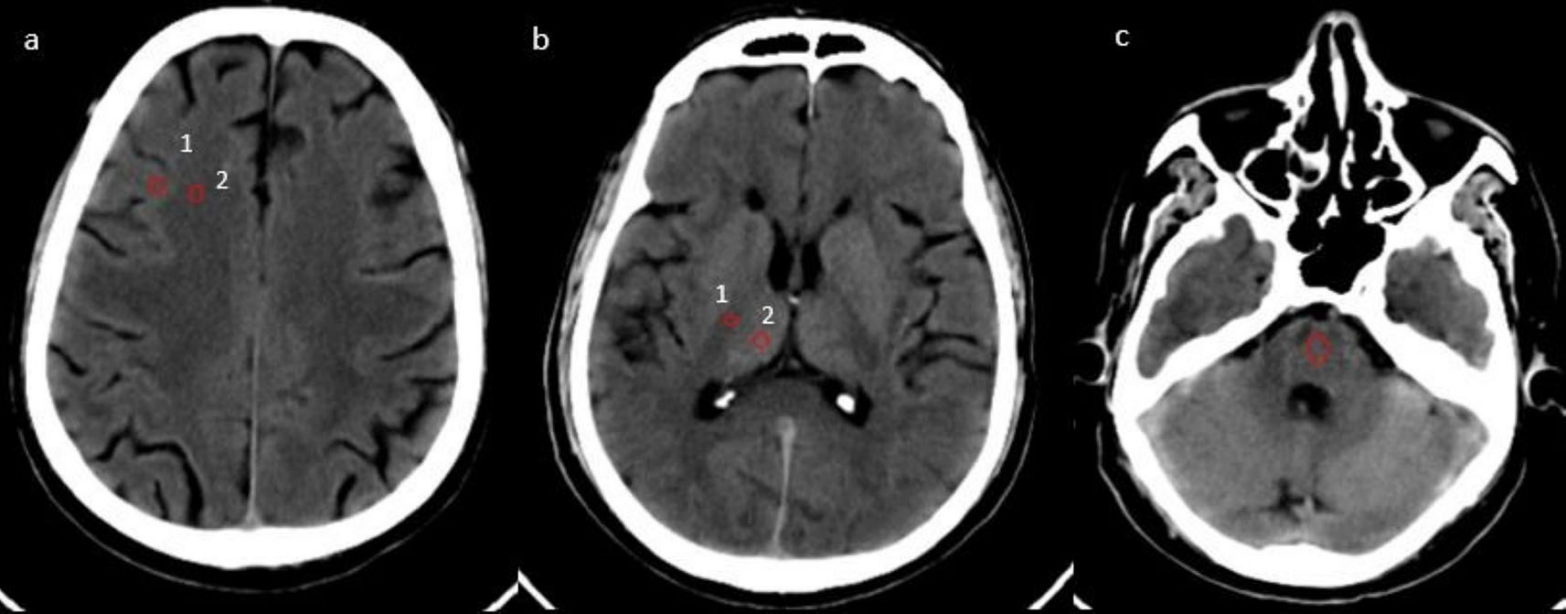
Axial CT images (DLIR-standard) of 61-year old male at the level of centrum semiovale (a) and basal ganglia (b) showing region of interest (ROI) in gray matter and white matter. Axial CT image at the posterior cranial fossa (c) with ROI drawn in the pons region between the petrous bone.
